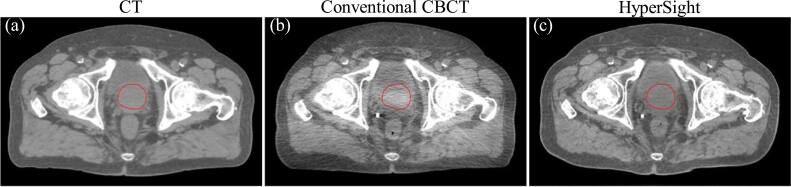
Comparisons of image quality between CT and CBCT acquired from Varian TrueBeam with conventional CBCT and HyperSight systems. (a), (b) show images from prostate treatment. The prostate is highlighted with red contours. The CBCT images for each treatment site were acquired from the same patient but different treatment fractions. All figures were prepared de novo for this review using institutional data.
